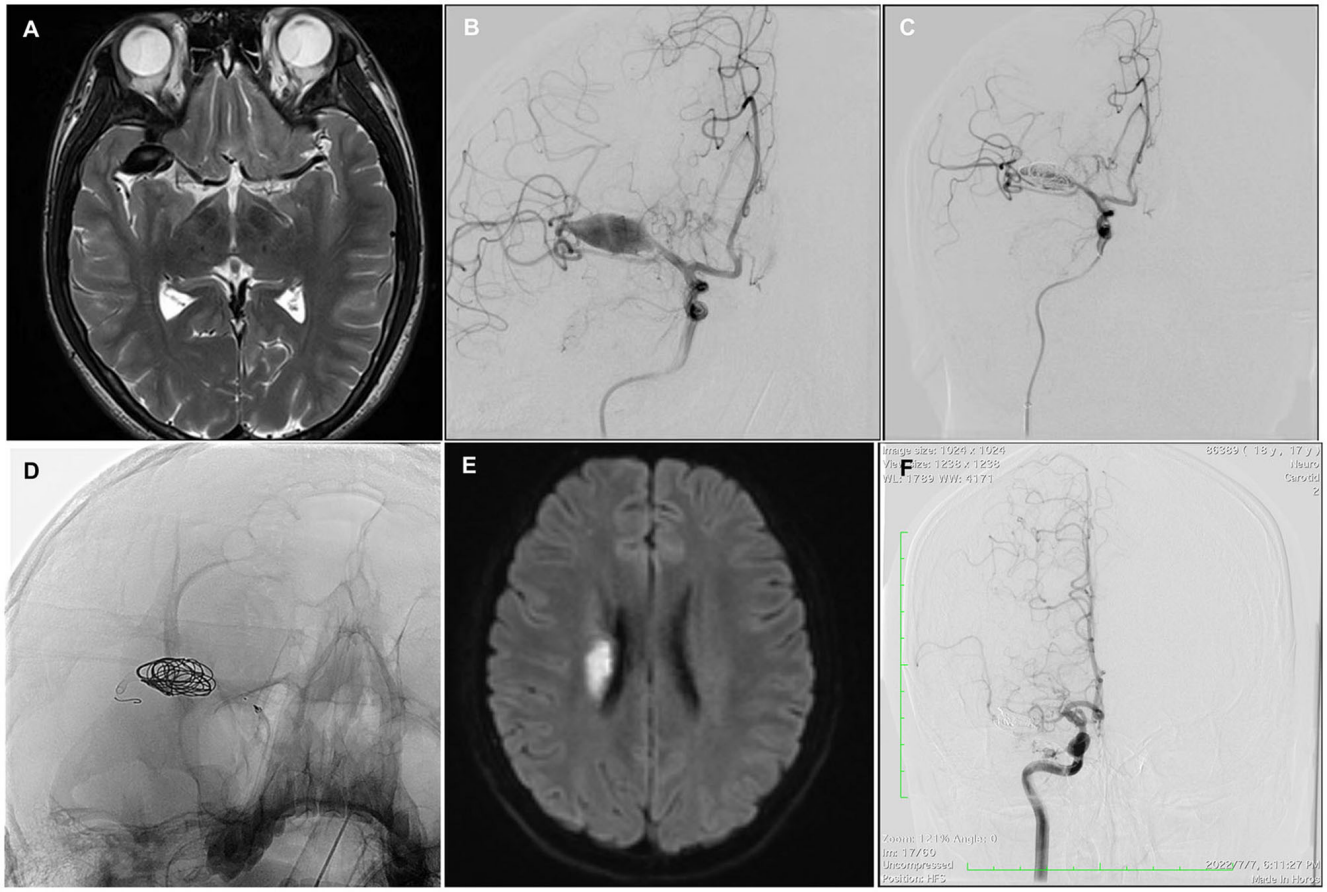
Case 6. A 16-year-old man with a giant fusiform right MCA M1 segment aneurysm, 26*12*11 mm in diameter (A, B). The aneurysm was treated with a single PED and adjunctive coil embolization (C, D); 6 h after the procedure, the patient developed an acute left hemiparesis. MR imaging showed acute infarction at the right corona radiata (E). Twelve-month follow-up angiography showed a complete occlusion of the affected vessel (F).
